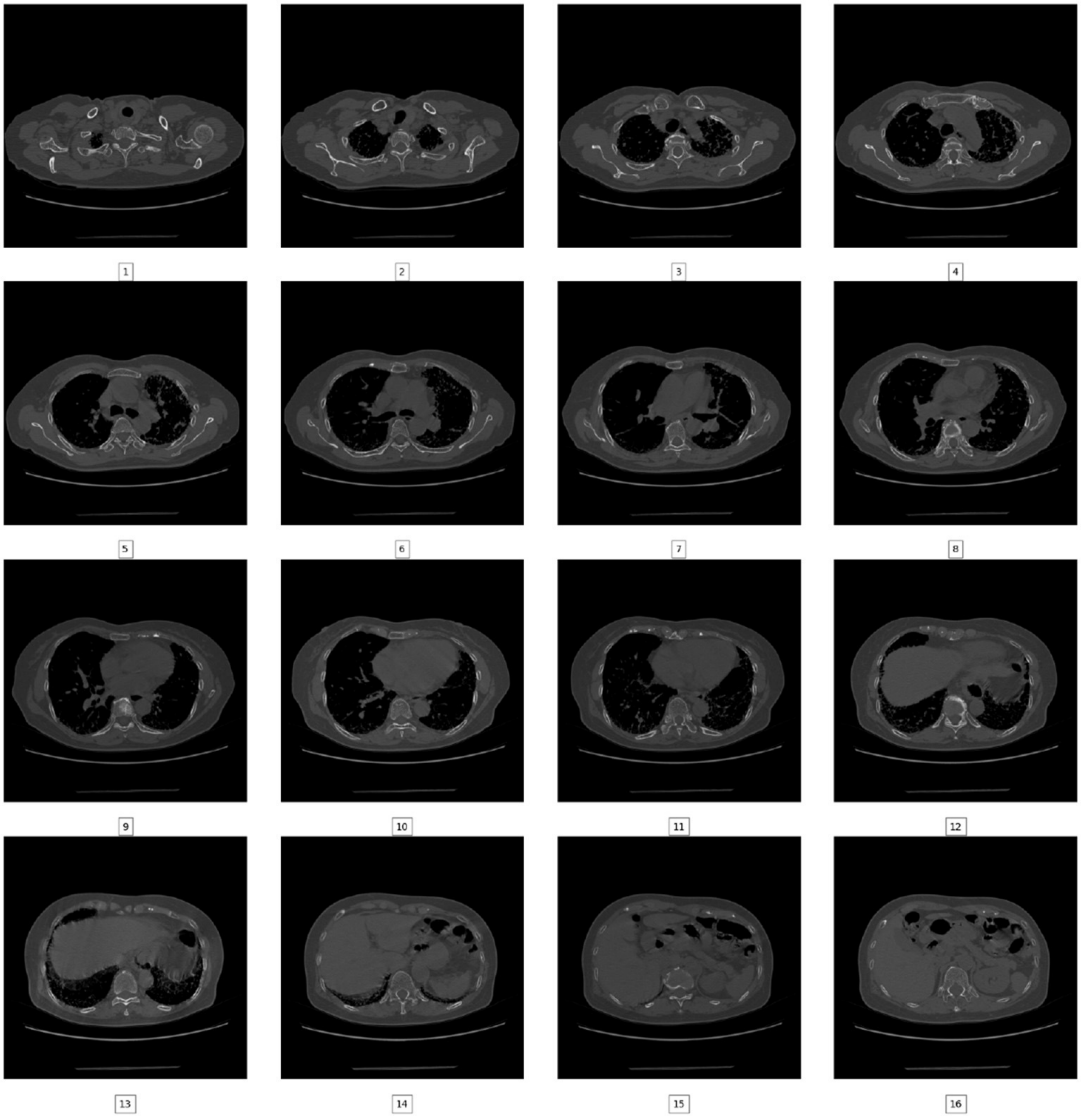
A Chest CT scan sliced into multiple images.
